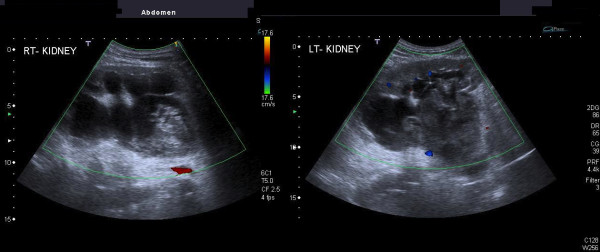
Ultrasound of renal pelvises demonstrating bilateral hydronephrosis and echogenic material.
